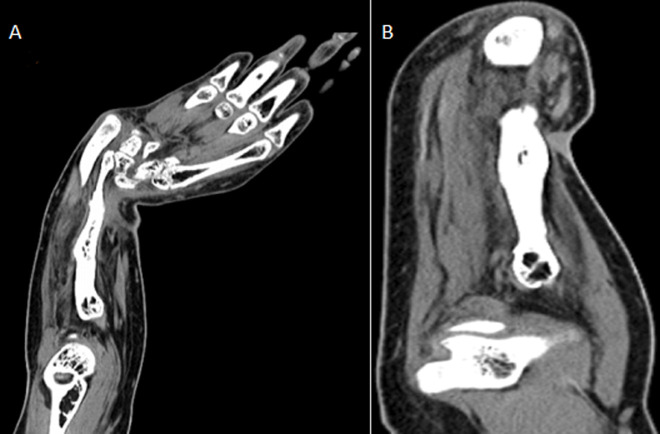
computed tomography coronal (A) and sagittal (B) view of right forearm showing non-visualization of the proximal 1/3^rd^ of the radius with the shaft of ulna appearing curved and poorly supporting the wrist joint; muscles of the forearm show disuse atrophy
